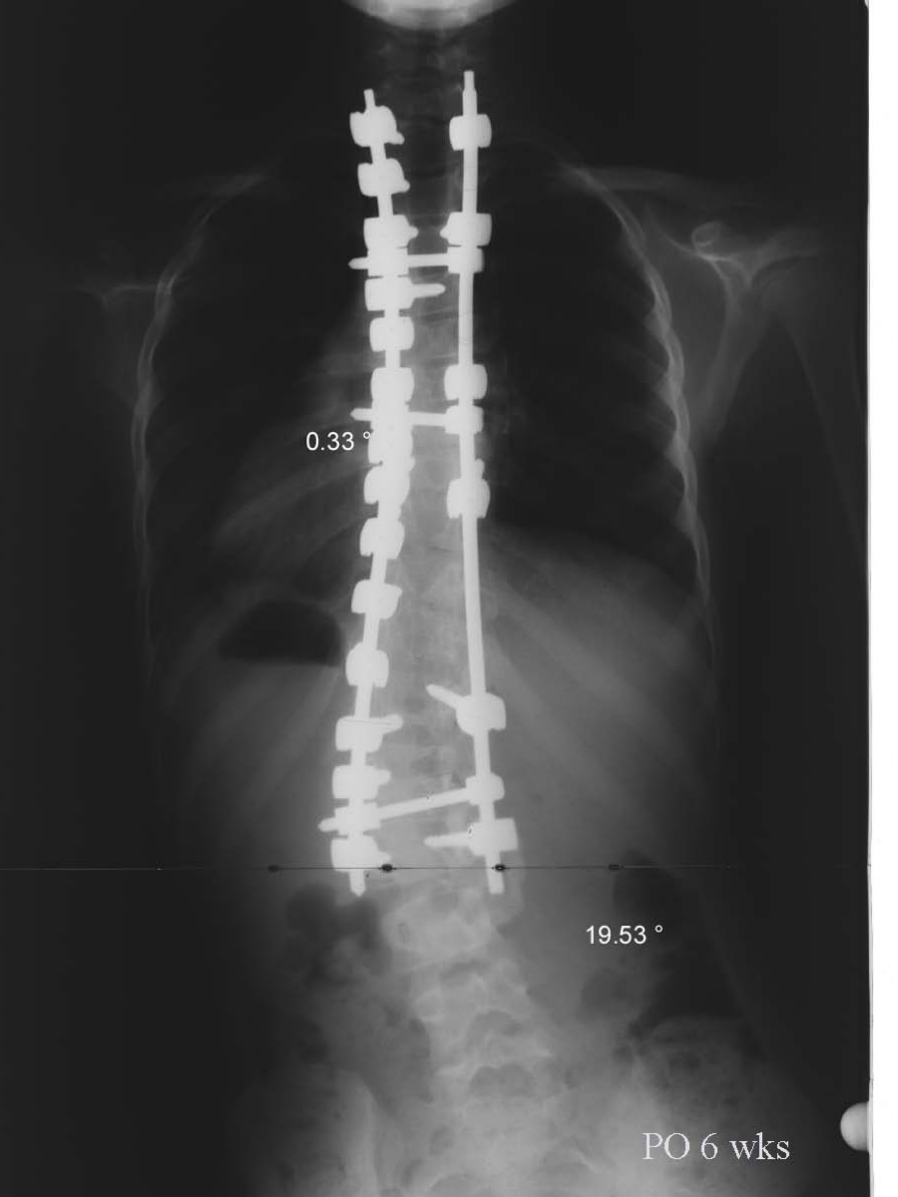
Anteroposterior radiograph of the same patient taken 6 weeks after surgery. The main thoracic curve was corrected to 0.3° and the lumbar curve was corrected to 19.5°.
